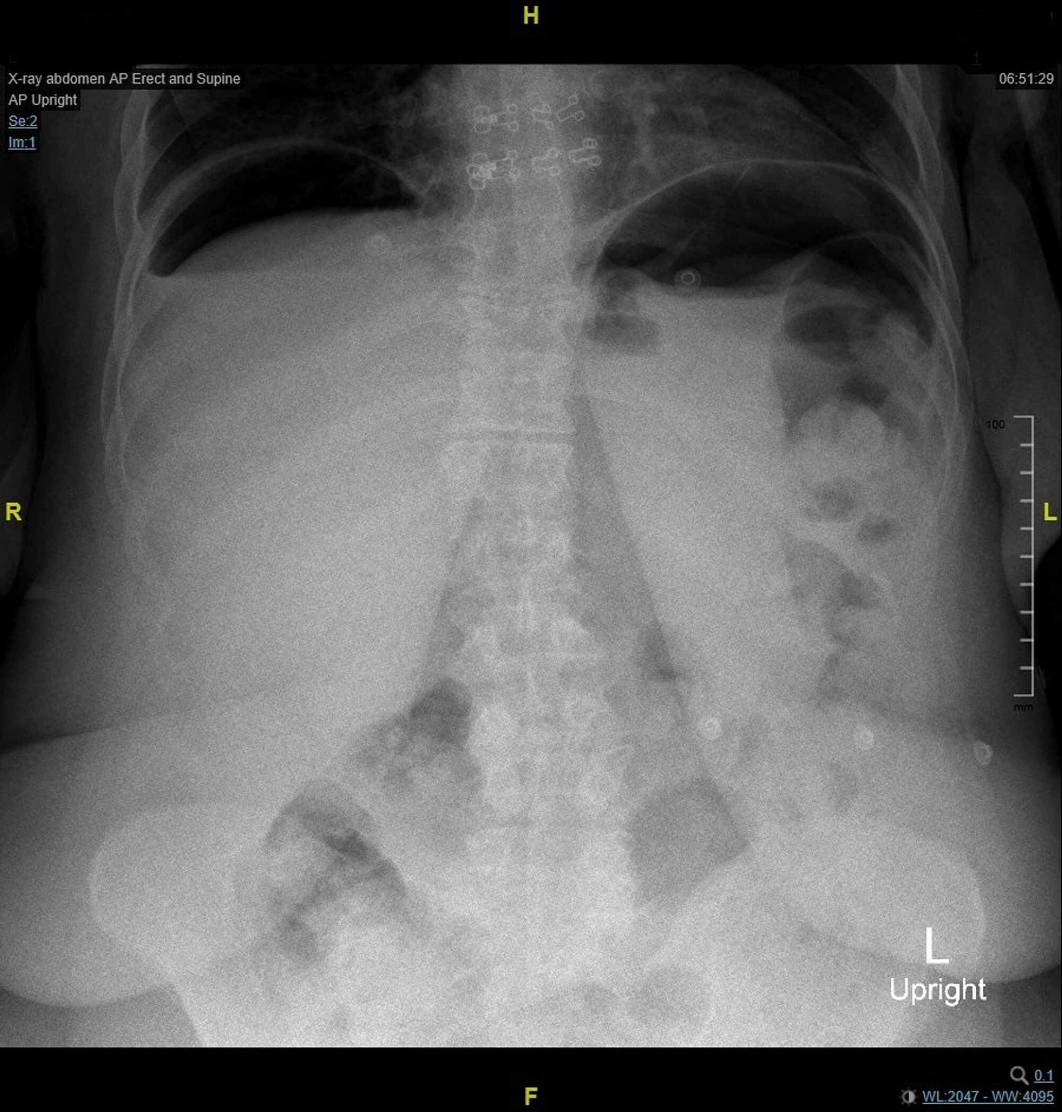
Erect abdominal X-ray showing air under diaphragm.
